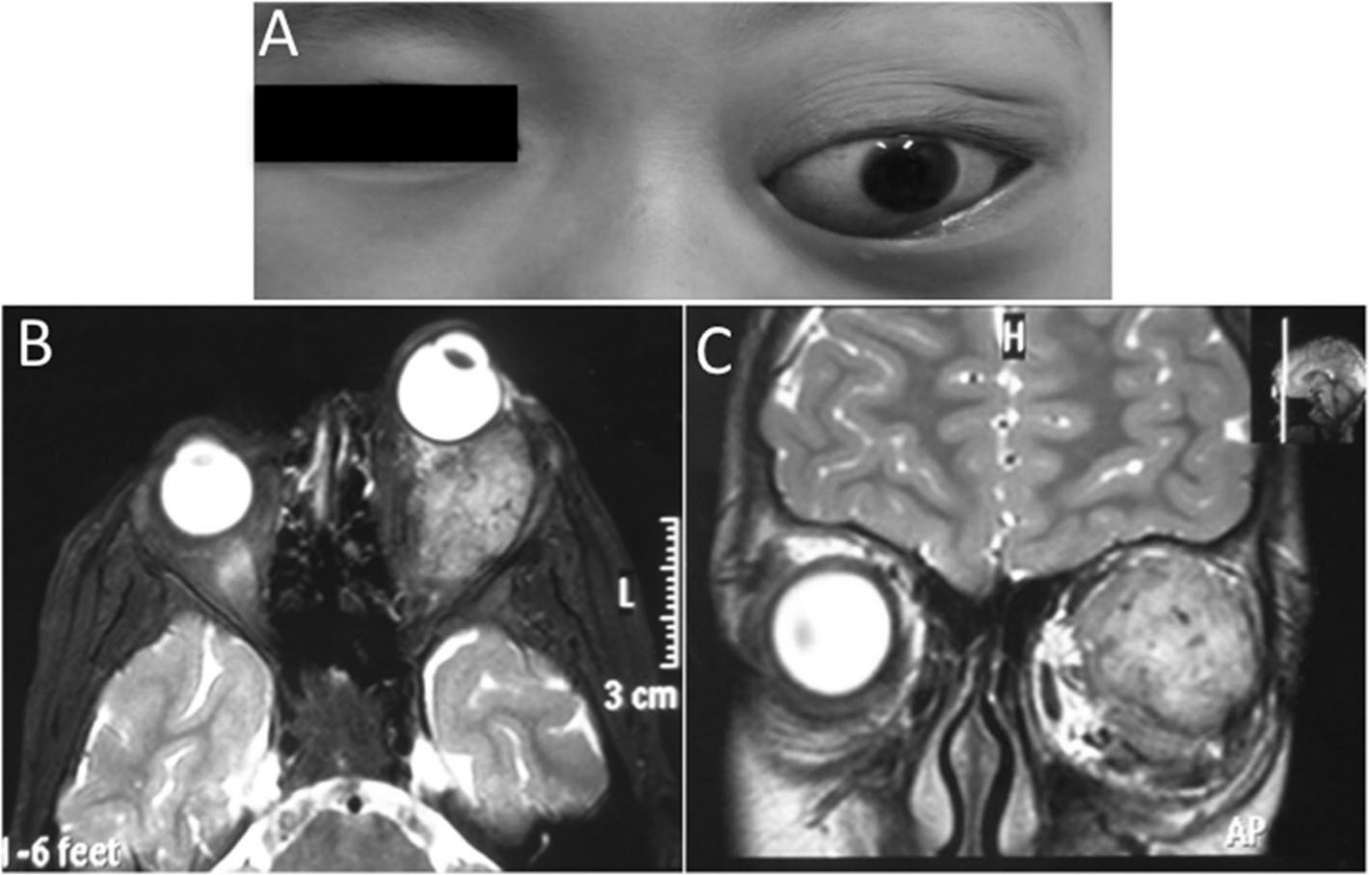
a Nonaxial proptosis of the left eye in patient 3, with downward displacement of the globe; b and c, T2-weighted axial and coronal series showing a well-defined recurrent mass with intermediate signal intensity in the posterosuperior orbit
